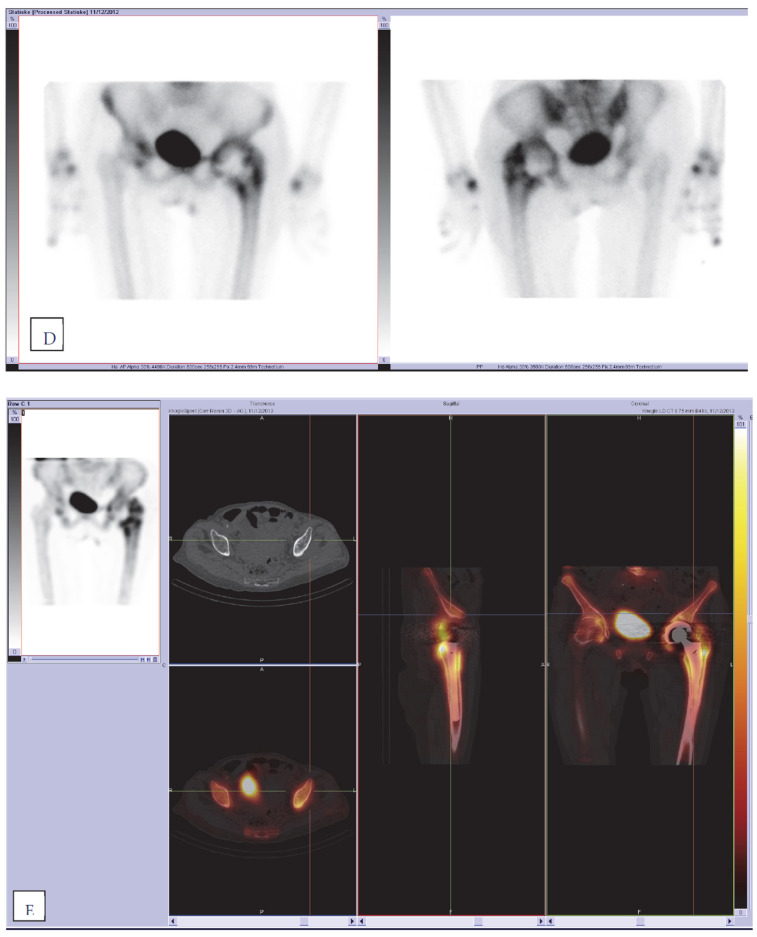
Three hybrid imaging modalities for the same patient with an infected hip prosthesis. (A-1) and (A-2) FDG PET/CT, showing high FDG uptake in the periprosthetic soft tissue communicating with intraarticular space (red arrows). (B,C) Combined In-labeled leucocyte SPECT/CT (B) and bone marrow SPECT/CT (C) (showing focal leucocyte uptake in the soft tissue (arrows) and mis-matched focal leucocyte uptake in the prosthesis bone interface in the femoral part (arrows). (D,E) Bone scan; static uptake (D), SPECT/CT (E) (showing generally high uptake in bone tissue around the prosthesis in proximal femur).
